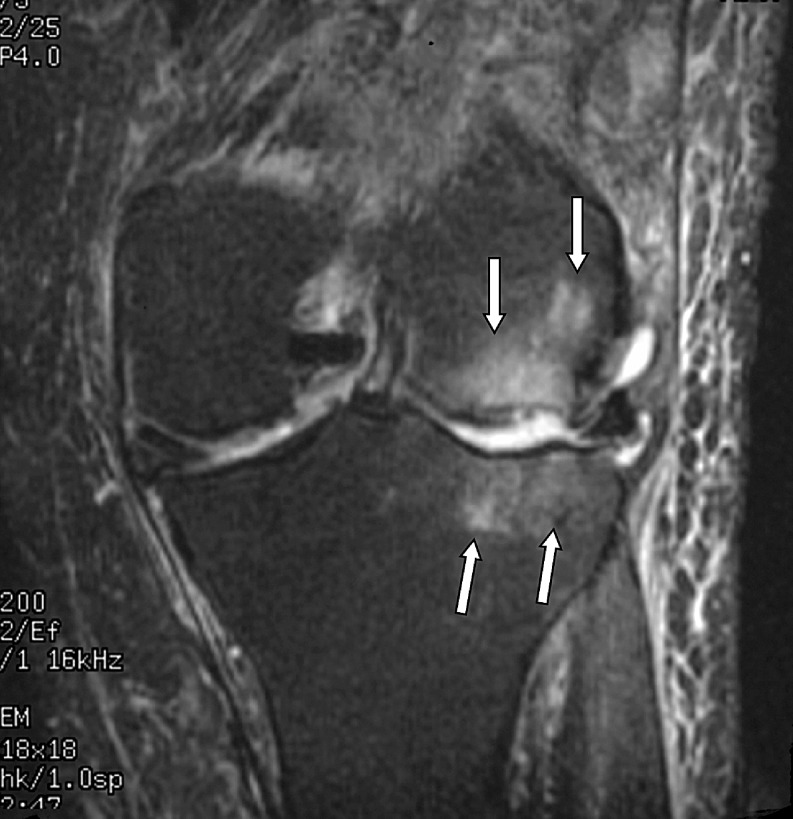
Global edema with a total knee joint replacement in a 72-year-old male patient. The radiograph demonstrated loss of cartilage joint space within the lateral compartment. Coronal fast fat-suppressed T2-weighted image (TR 3200/TE 72/256 × 192) shows extensive edema in the femoral and tibial condyles (*arrows*)
